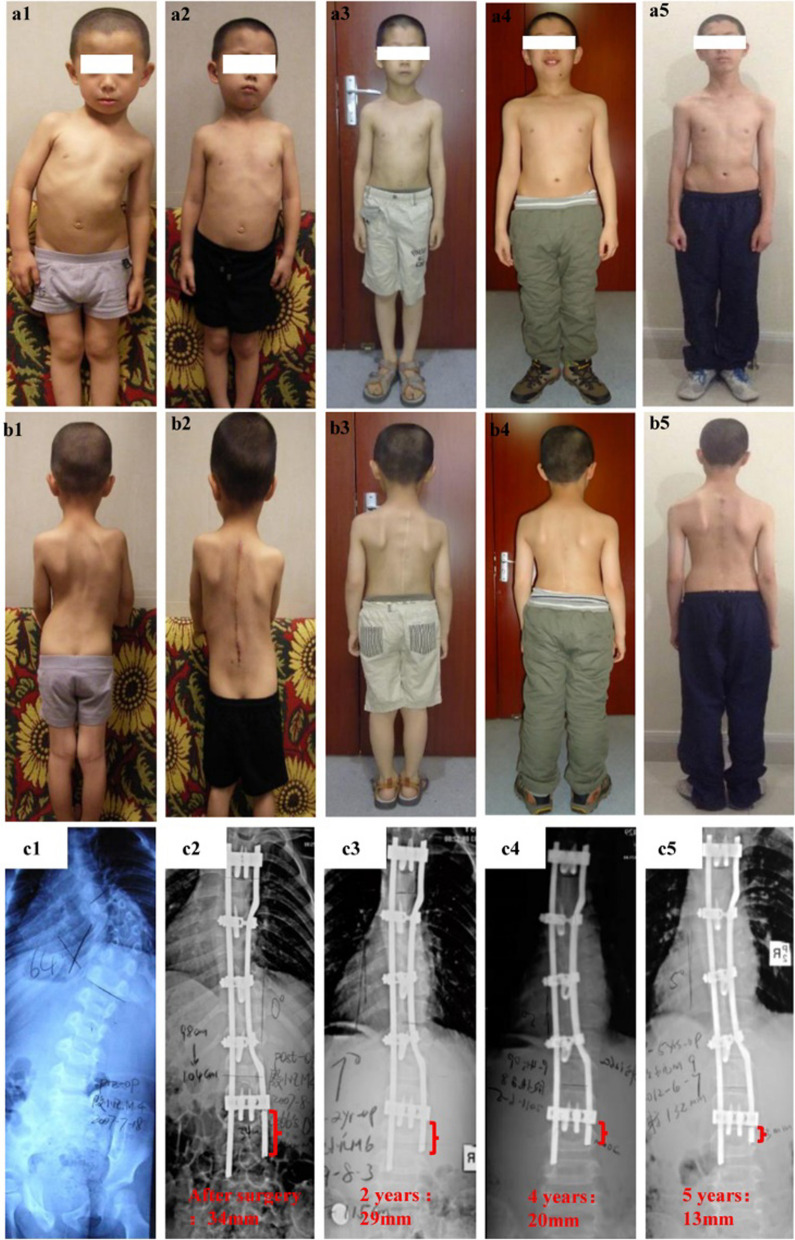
Immediate and ongoing correction of early onset scoliosis (EOS) after treatment with the plate-rod spinal system. a1, b1, c1, d1 A 4-year-old boy with a preoperative Cobb angle of 64°. a2, b2 Postoperative photographs. The postoperative radiograph c2, d2 shows a Cobb angle of 0° degrees and a rod tail reserve length of 34 mm. a3, b3, c3, d3 Photographs and radiograph at postoperative 2 years. The rod tail length is 29 mm. a4, b4, c4, d4 Photographs and radiograph at postoperative year 4. The rod tail length is 20 mm. a5, b5, c5, d5 Photographs and radiograph at postoperative year 5. The rod tail length is 13 mm
